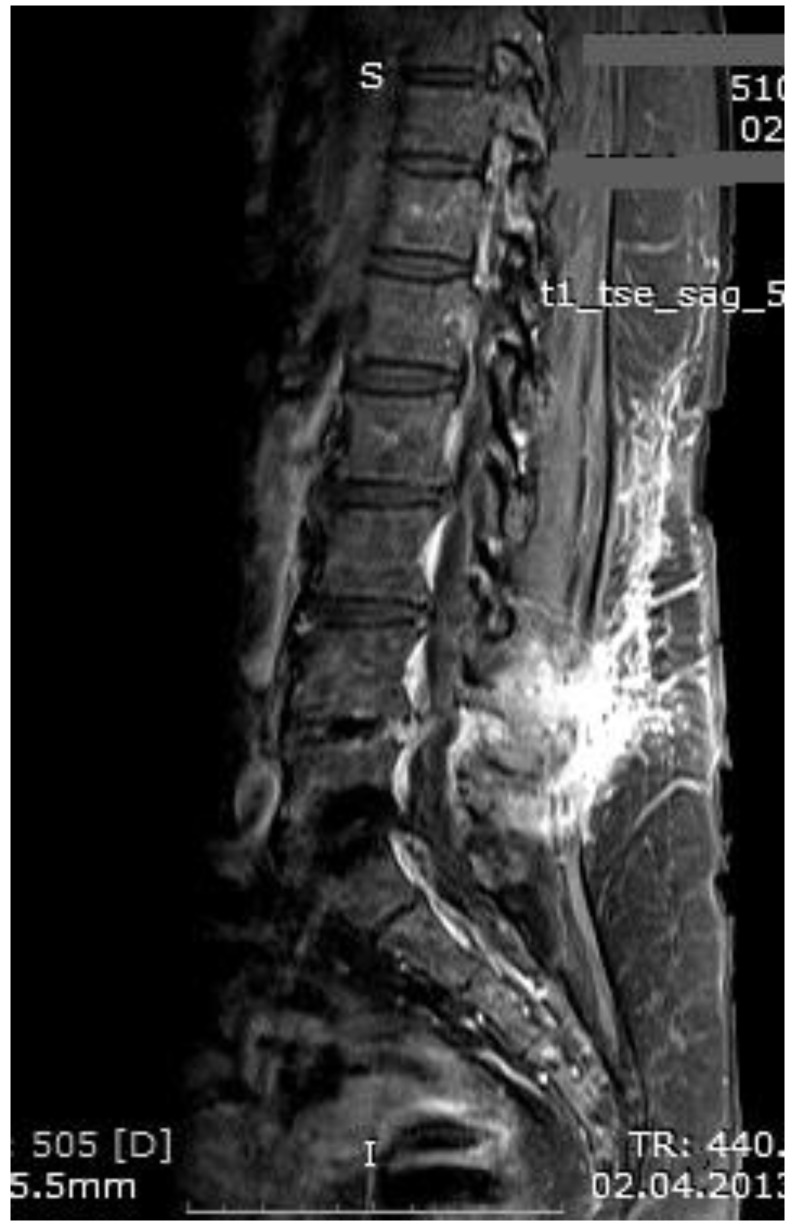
MR imaging T1-weighted scan showing inflammatory infiltrates in the course of pre-HBO_2_ treatment.
